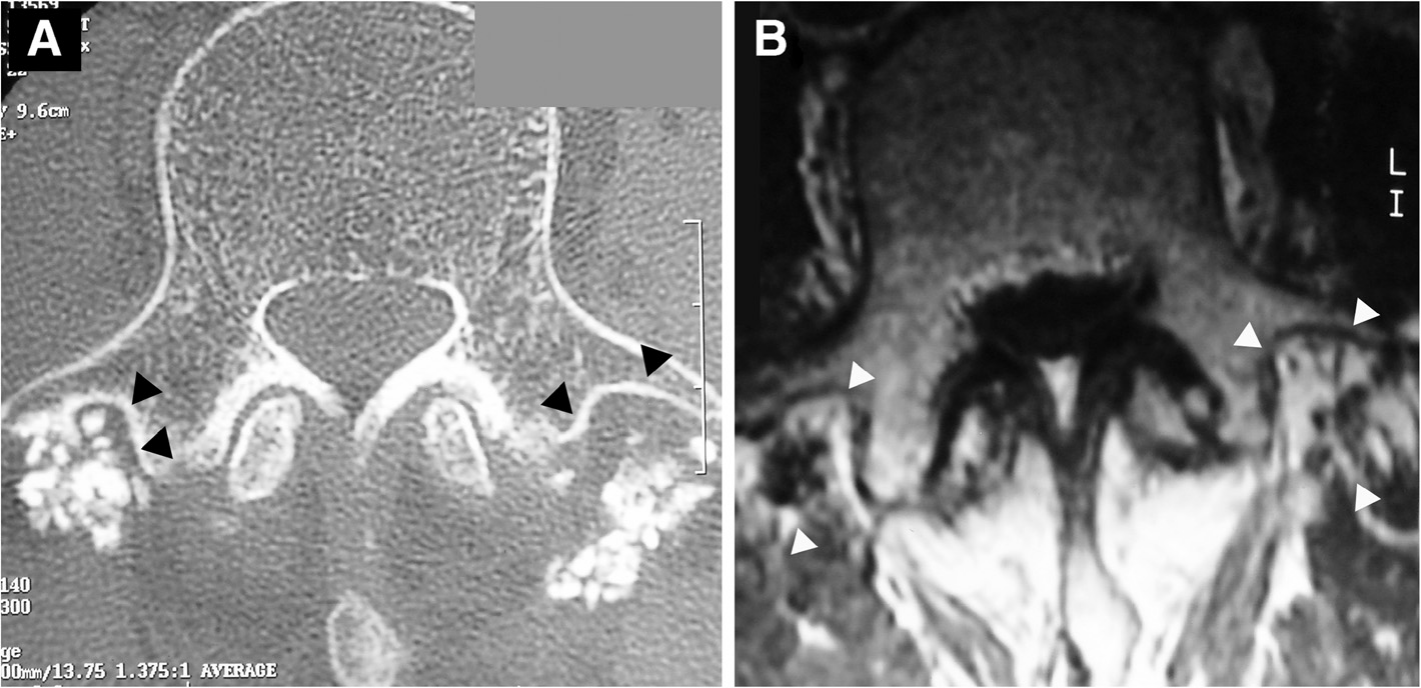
Preoperative imagings A; Computed tomography of L4 showing scant bone formation around the granulation tissue and few bony intertransverse bridges between the transverse process and HA. Because of intervening fibrous tissue, contact between HA and the vertebrae is limited (arrowheads). B; Gadolinium-enhanced magnetic resonance imaging (MRI) of L4. Enhancement was observed around transplanted HA (arrowheads), suggesting failure of the posterolateral fusion.
